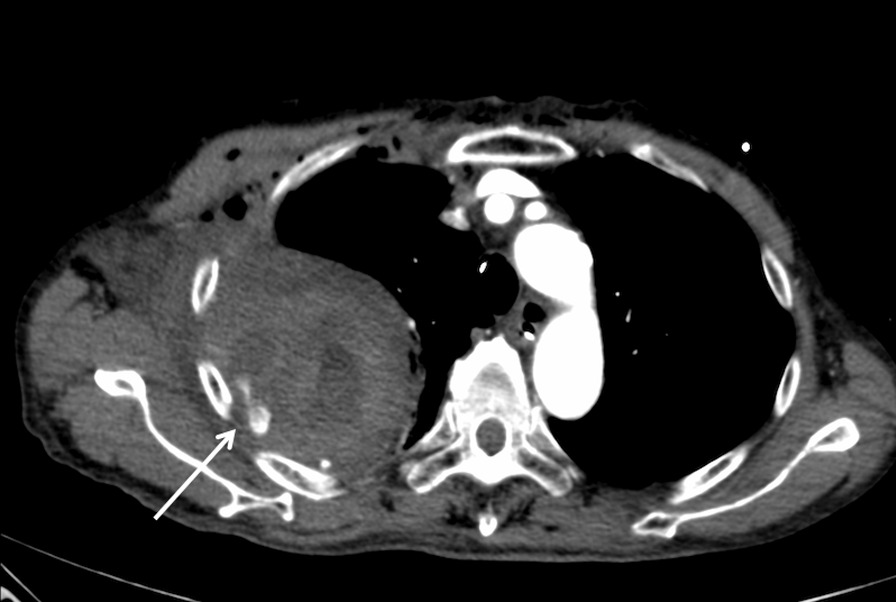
Contrast enhanced computed tomography showed hemothorax with bleeding from the right fourth posterior intercostal artery (arrow)
